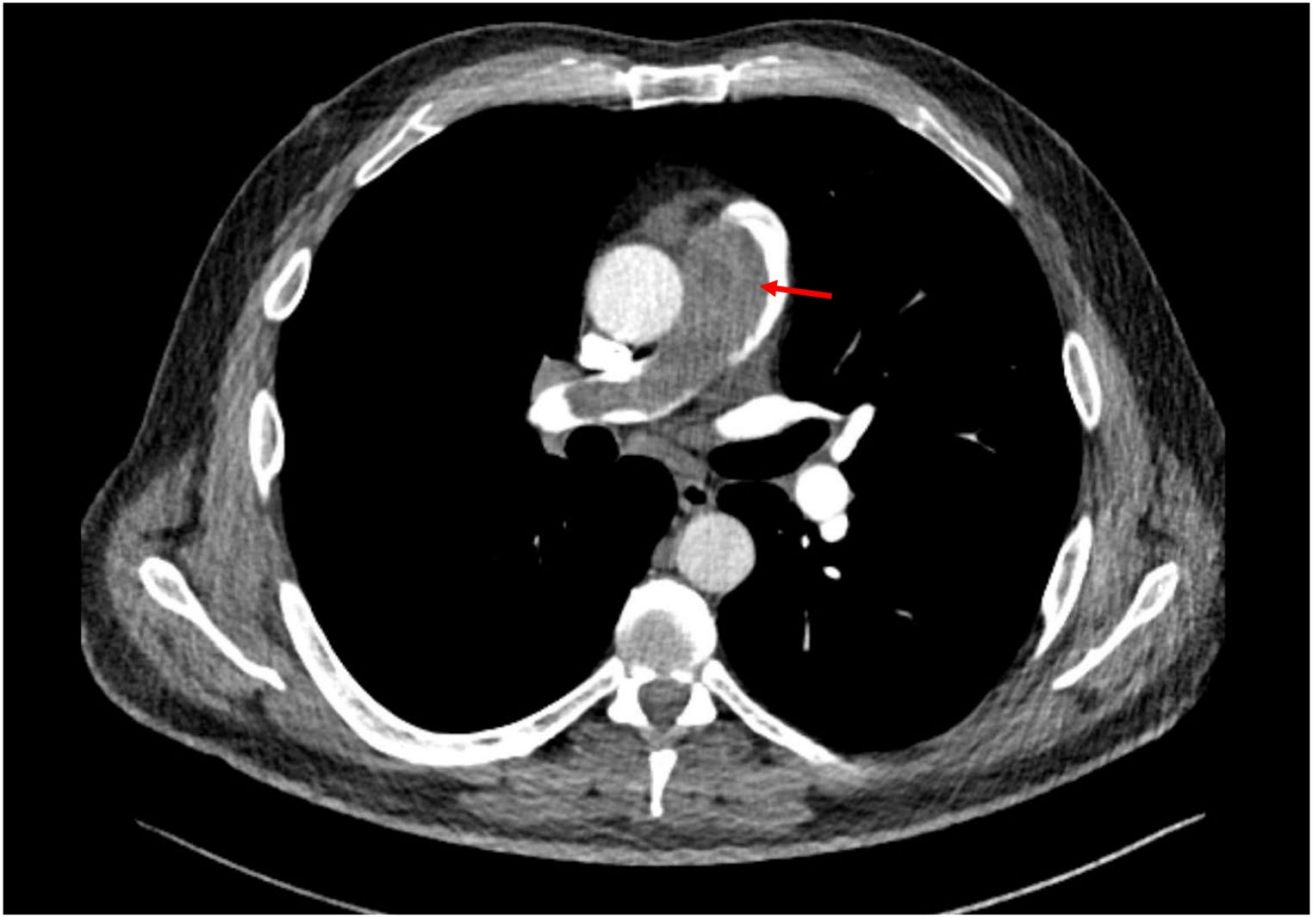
CT-angiography showing a bilateral pulmonary artery embolism with large central thrombus (red arrow) in the pulmonary trunk
